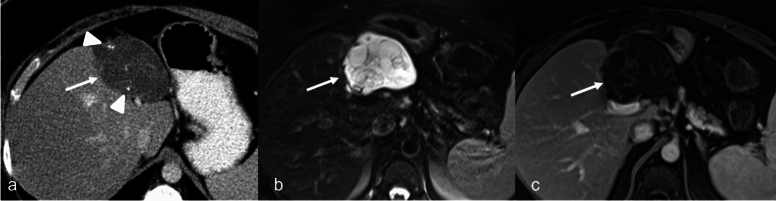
Mucinous cystic neoplasm of the liver in a 59-year-old female patient with a history of endometrial cancer and a liver lesion noted on staging CT. Axial CT (a) shows a lobulated cystic lesion with septations and small areas of calcifications (arrowheads). Axial MRI T2-weighted fat-saturated (b) and postcontrast T1 (c) images demonstrate a cystic lesion with multiple septations, seen as linear hypointensity within in the cyst (arrow). The septations demonstrates smooth enhancement post contrast and no evidence of nodularity
